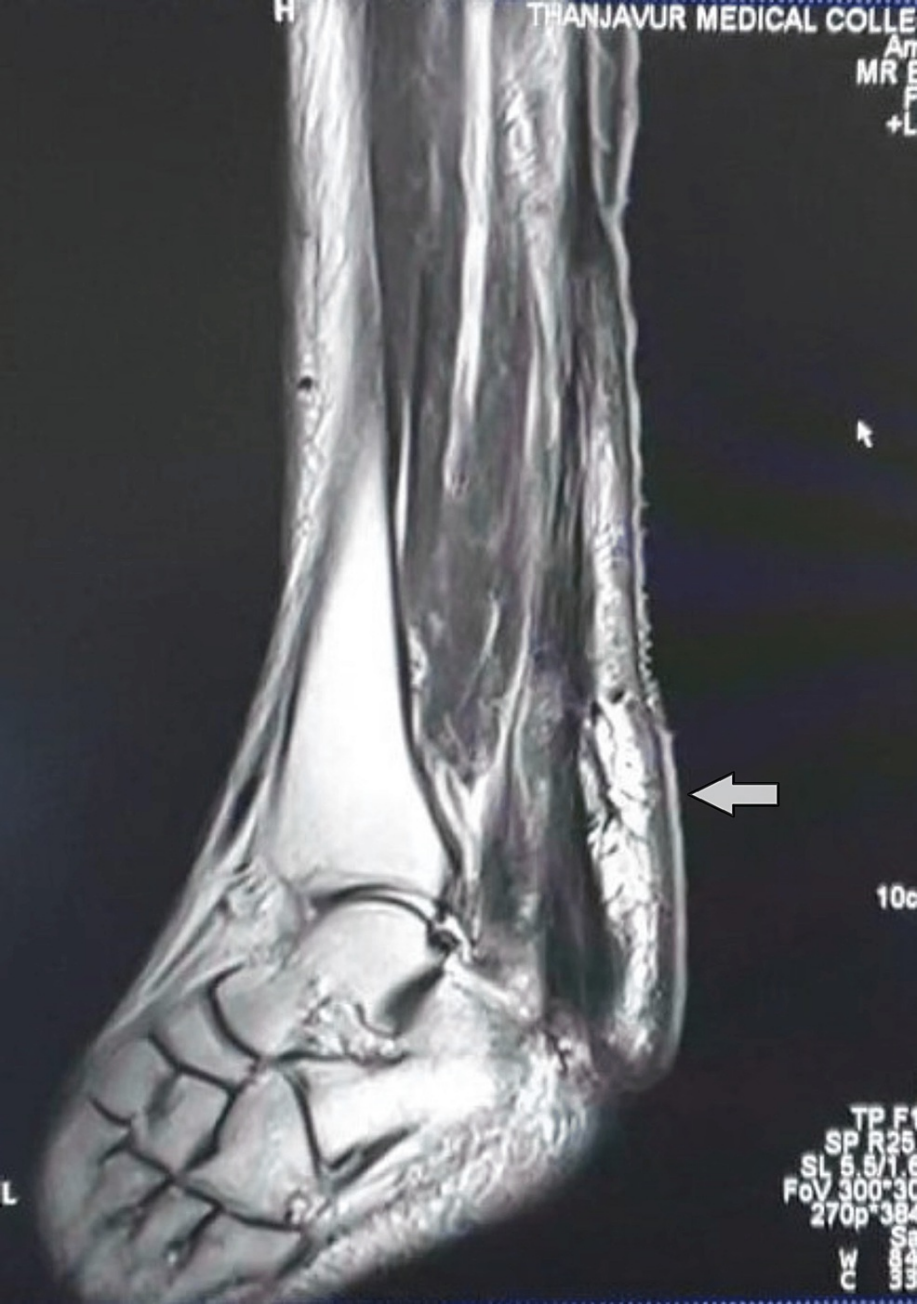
MRI image of the left ankle in case 2 MRI sagittal T1 image revealed tendon xanthoma in tendo achilles of left side (arrow)
